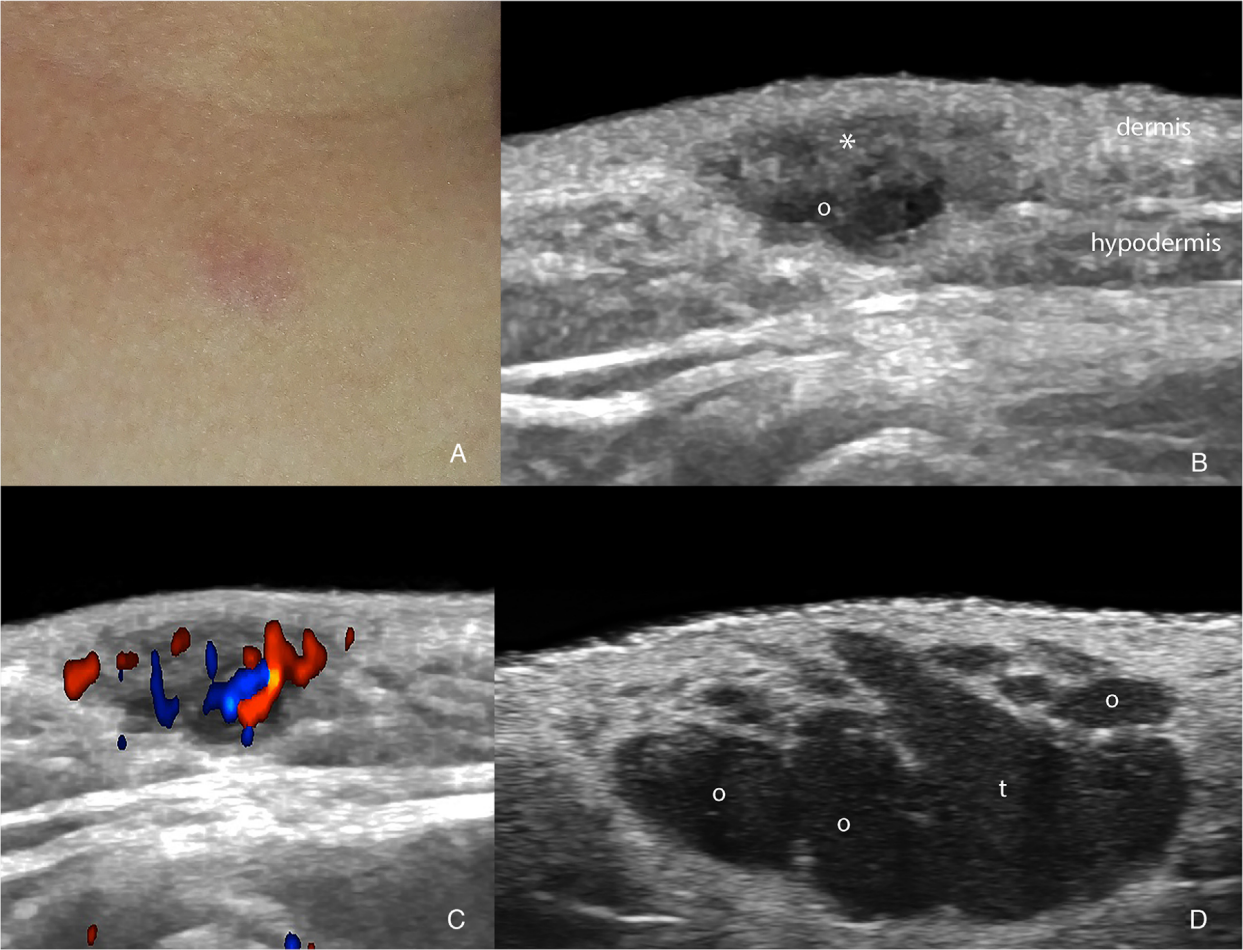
Nodular pseudolymphoma. Clinical‐ultrasonographic correlation. (A) Clinical image (6‐year‐old female; presternal region). (B–D) Ultrasonographic images. B and C at 18 MHz (B. grayscale and C. color Doppler) and (D) grayscale at 71 MHz demonstrate a slightly fusiform hypoechoic dermal and hypodermal structure (*) with a softly rising of the epidermis. On color Doppler, there is hypervascularity within the lesion. At 71 MHz, it is possible to better define the globules (o) and teardrop (t) signs at the lesion's bottom part
